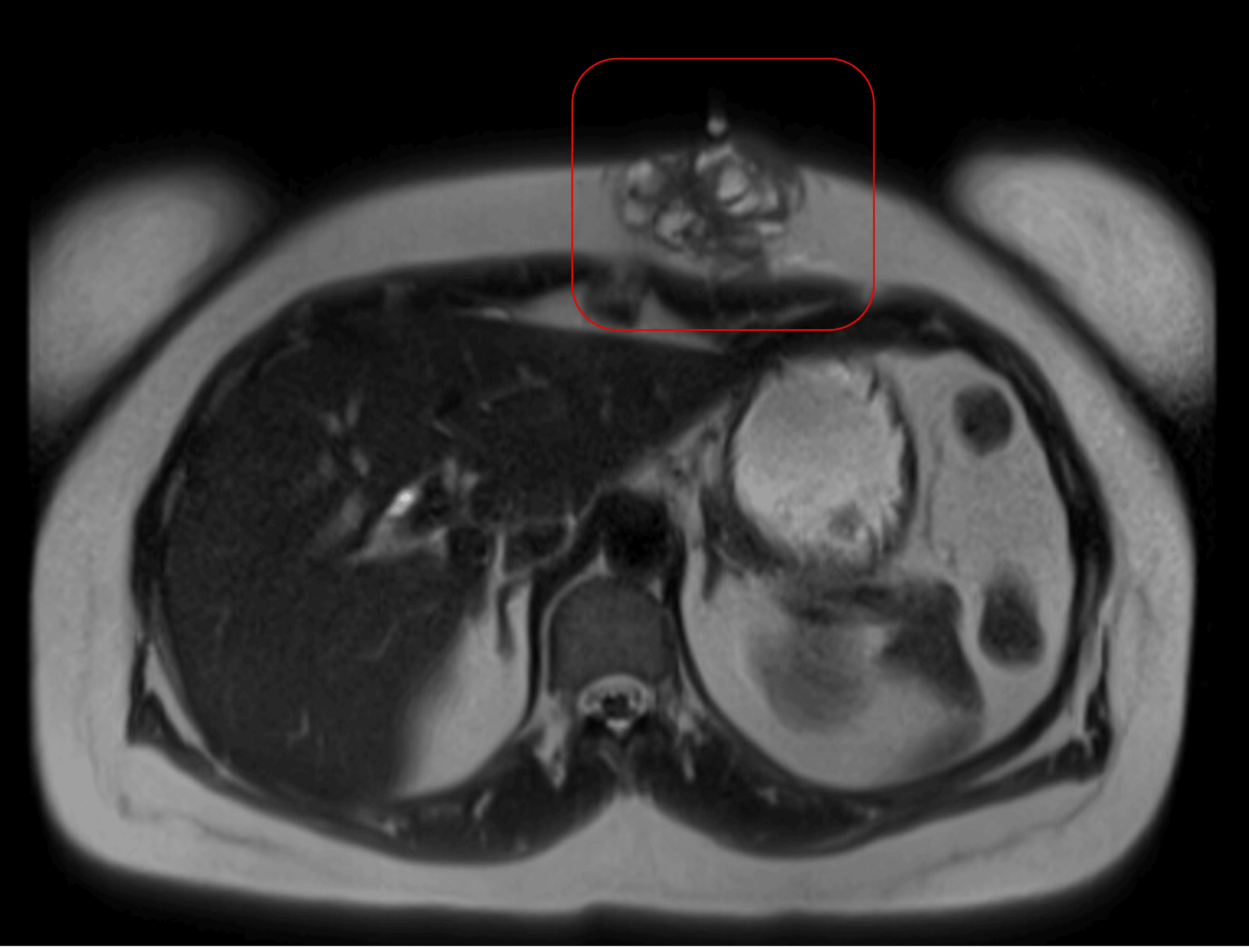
Axial T2-weighted MRI scan of the abdomen showing a subcutaneous enhancing mass (red square) in the left upper anterior abdominal wall.
